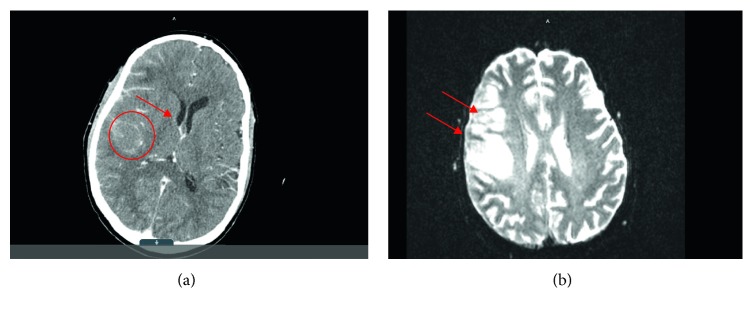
(a) Initial CT scan showing increased diffuse enhancement of right cerebrum as well as ill-defined hypodensity in the posterior right frontal lobe (circle). Slight mass effect is shown upon right lateral ventricle with edema (arrow). (b) MRI brain with gadolinium showing parenchymal and leptomeningeal enhancement over the right frontal and temporal lobes with associated T2/FLAIR signal involving right frontal lobe cortex (arrows), finding suggestive of meningoencephalitis.
